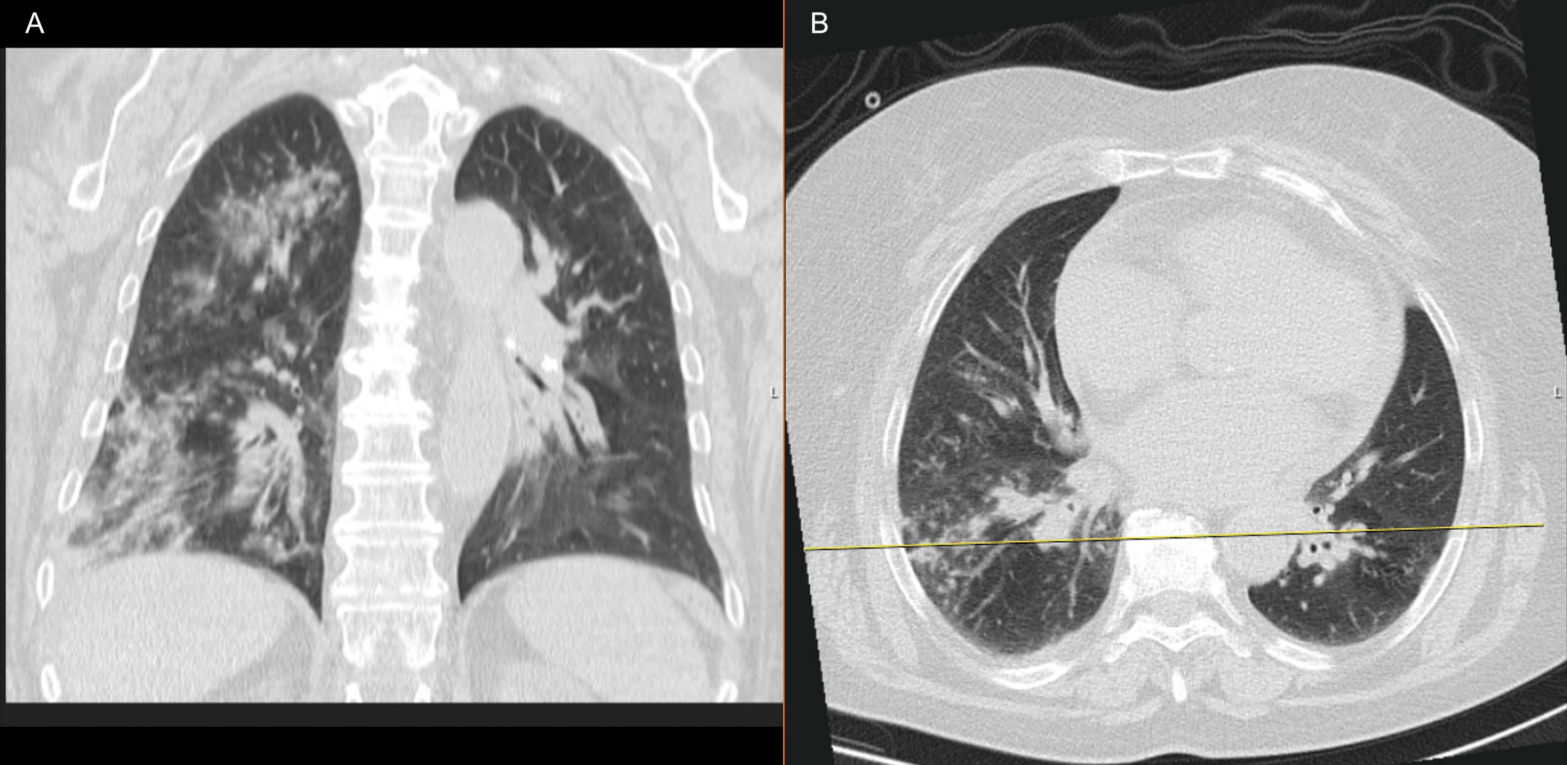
Computed tomography of the chest: coronal view (panel A) and axial view (panel B). Consolidation in the right upper, middle, and lower lobes is associated with mucoid impaction in the small airways of the latter. Subpleural opacities in the posterior fields of both lungs. Small bilateral pleural and pericardial effusions.
